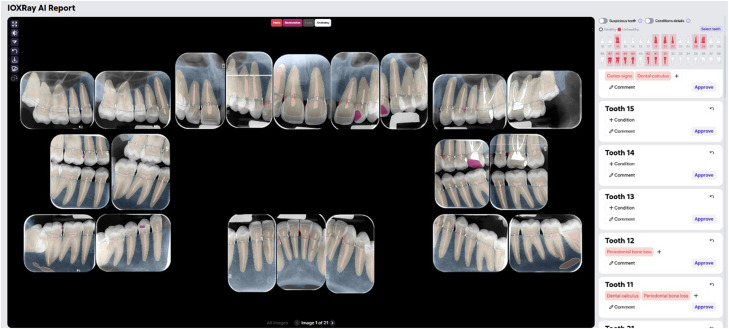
Screenshot of the interface for interpreting full-mouth series (FMS) radiographs in AI software.
